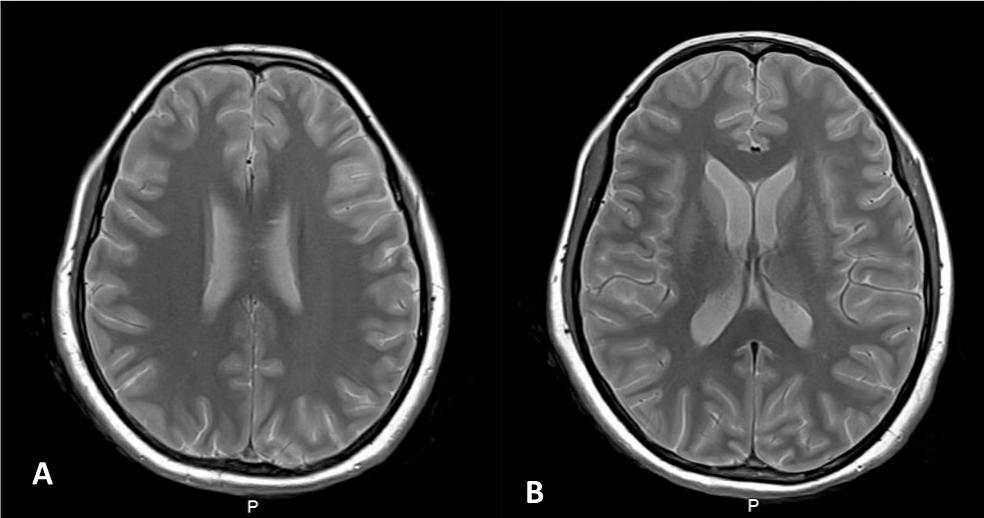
Cranial MRI scan on the fourth day of hospitalization in different cuts (images A and B). Both images reveal T2 hyperintensity in some sulci, leptomeningeal enhancement, and ventricular enlargement (more visible in image B).
